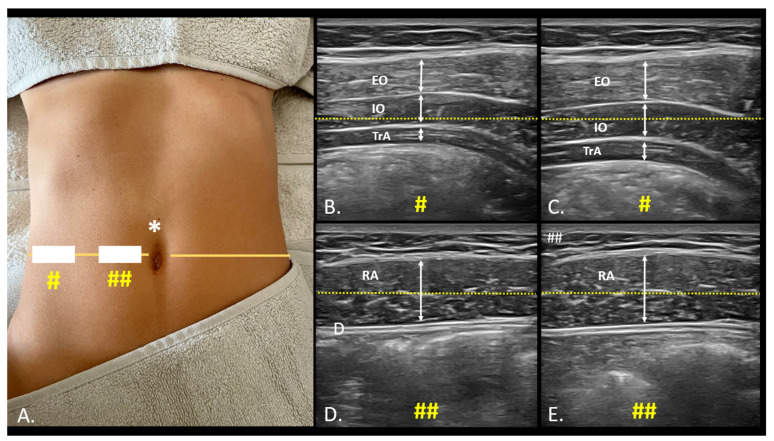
USI assessment and measures of anterolateral abdominal wall. Placement of the probe for the evaluation of the lateral muscles of the abdomen (#) and the rectus abdominis (##) using the umbilical line (*) as a reference (dotted lines) (A); Evaluation of changes in ultrasound thickness between rest (B,D) and muscle contraction (C,E) of the lateral muscles of the abdomen ((B,C), #) and the rectus abdominis ((D,E) ##). Abbreviations: EO, external oblique; IO, internal oblique; RA, rectus abdominis; TrA, transversus abdominis.
